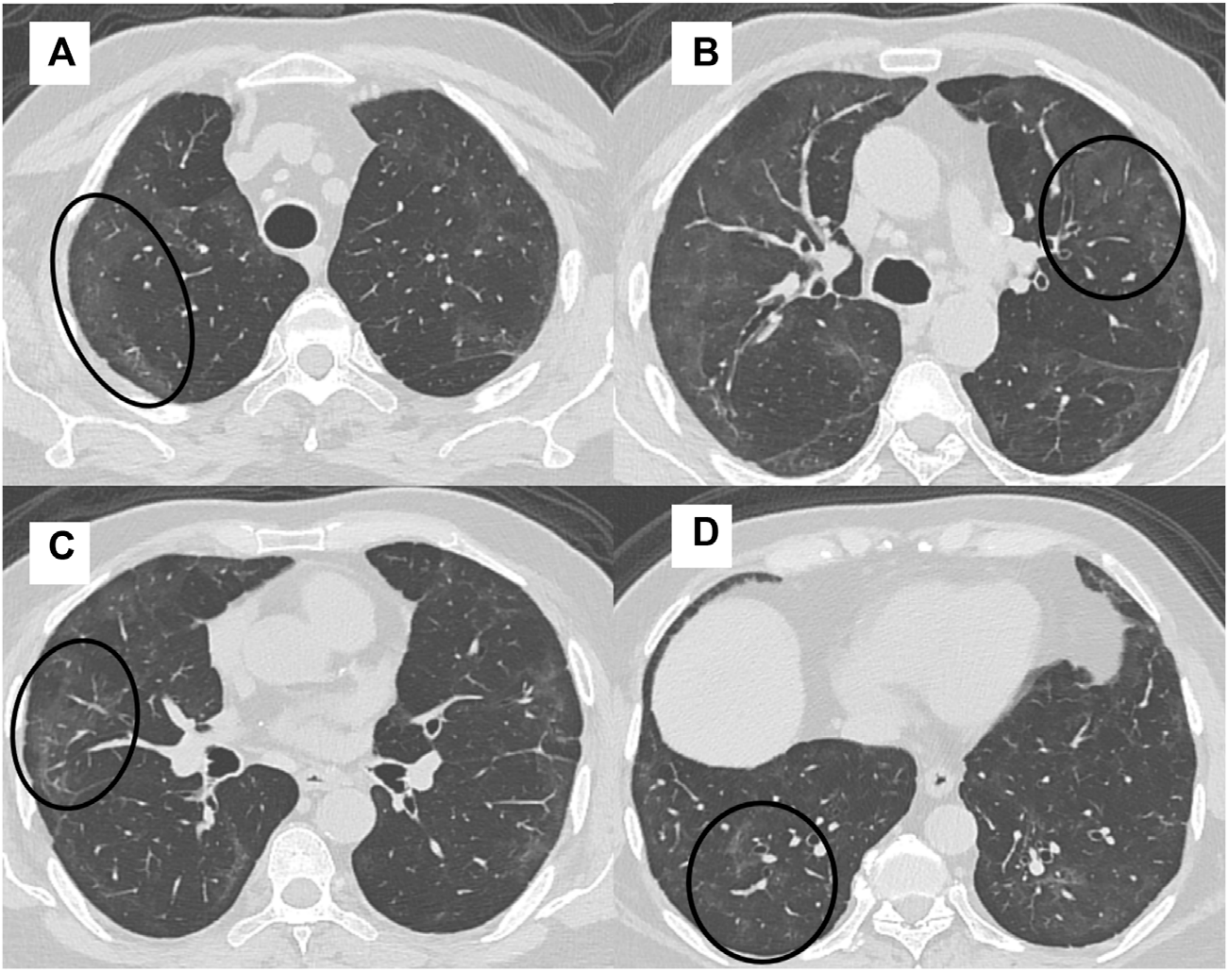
A 41-year-old man with intermittent cough, fatigue, and mMRC class 1 dyspnea had an FVC of 78% at 6 month follow up after COVID-19 ARDS. High-resolution CT (HRCT) chest axial sections (A–D) showed multifocal areas of GGOs in both lungs (black circles).
